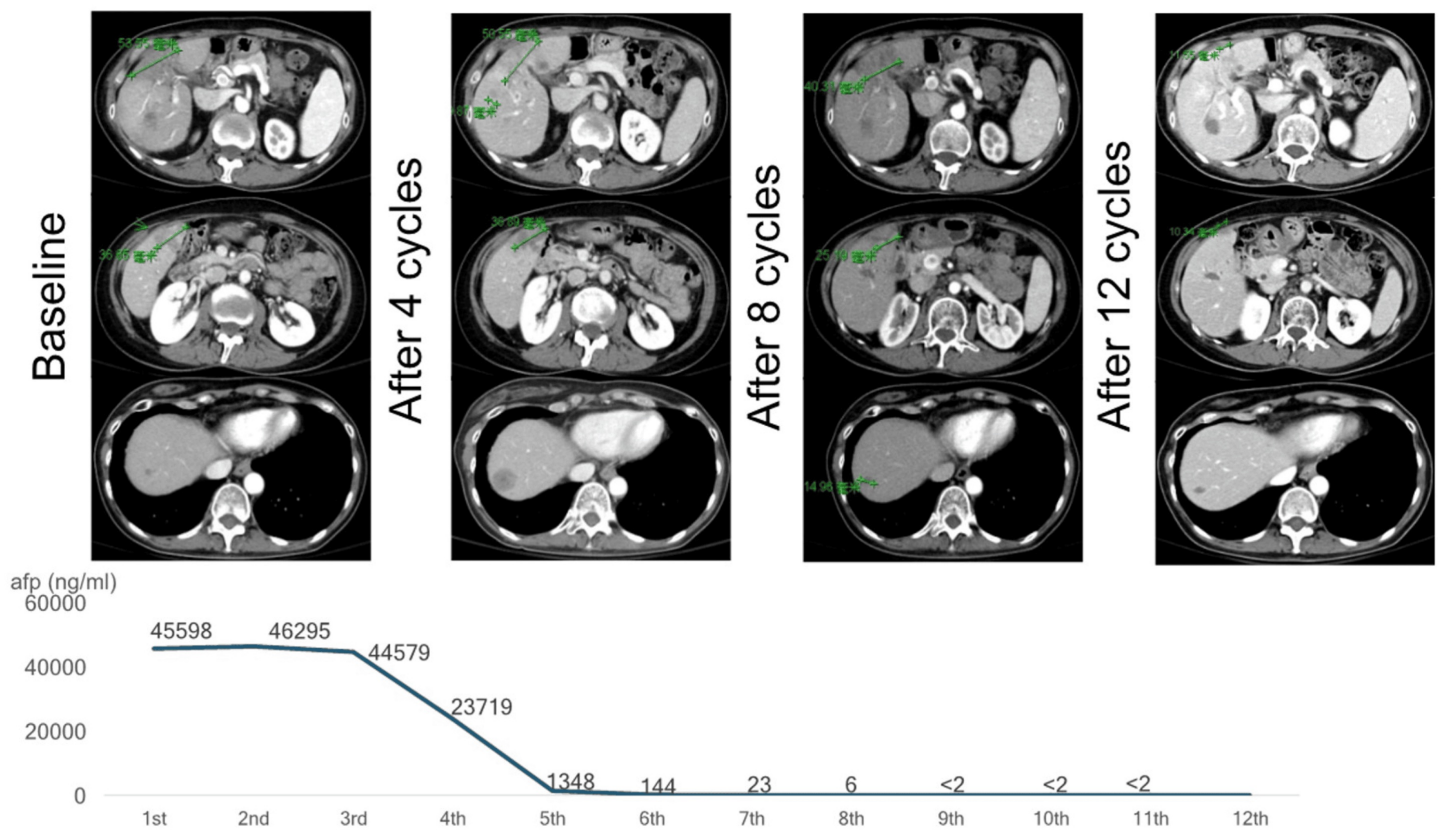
Patient serum AFP trends and CT imaging while on atezolizumab and bevacizumab AFP: alpha-fetoprotein
